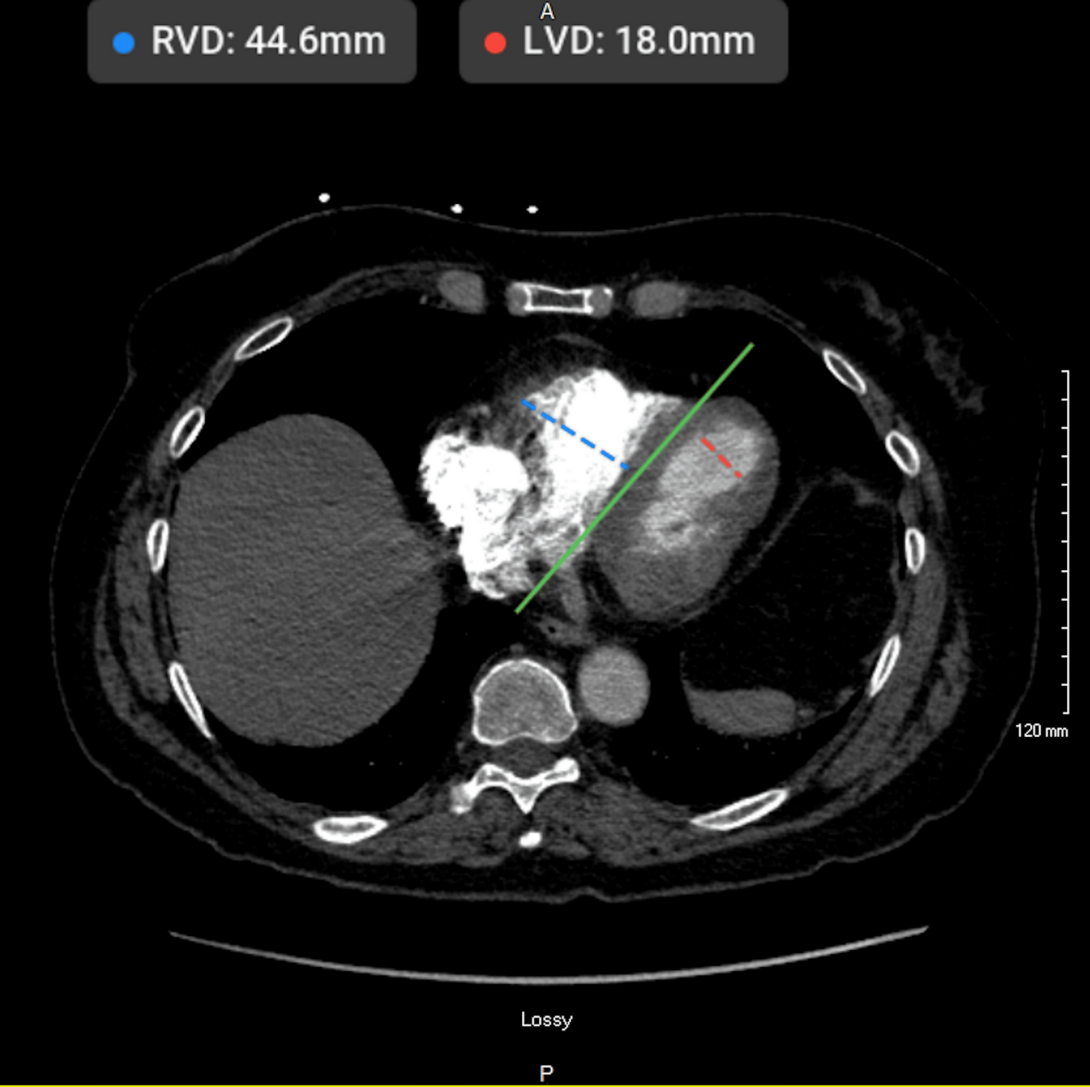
CTA chest showing a dilated right ventricle (blue dashed line, 44.6 mm) and a smaller left ventricle (red dashed line, 18.0 mm). The green line marks the interventricular septum. CTA: computed tomography angiography.
